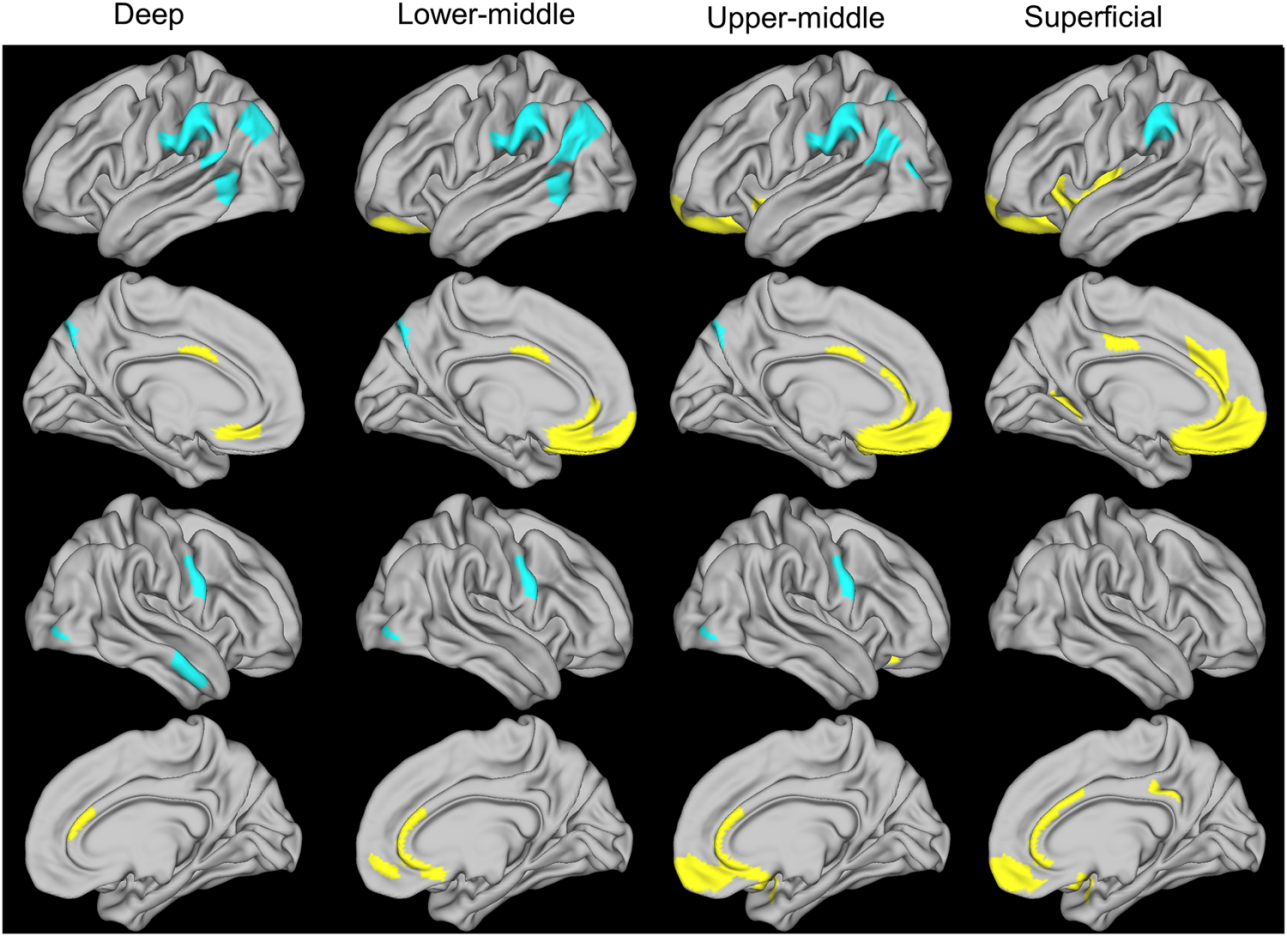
Relationship between worse ADI and cortical microstructure assessed as the T1w/T2w ratio at four cortical ribbon levels. The four cortical ribbon levels were as follows: deep, 5%–25% of cortical thickness from white-pial boundary; lower-middle, 30%–50%; upper-middle, 55%–75%, superficial, 80%–100%. Worse ADI was associated with increased T1w/T2w ratio (yellow) in medial prefrontal and cingulate regions, and decreased T1w/T2w ratio (blue) in supramarginal, middle temporal, and primary motor regions (*p* < .001) (*n* = 92). ADI area deprivation index, T1w/T2w, T1-weighted/T2-weighted.
